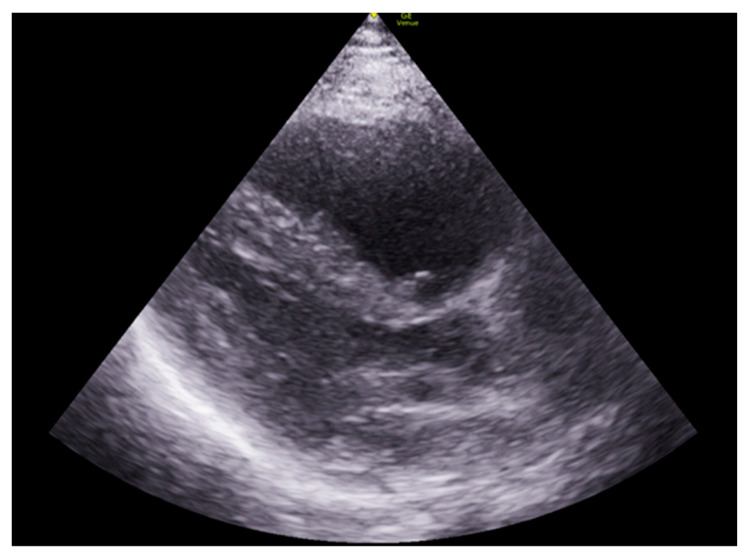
Parasternal long axis view depicting dilation of the right ventricle (image courtesy of S. Bezati).
